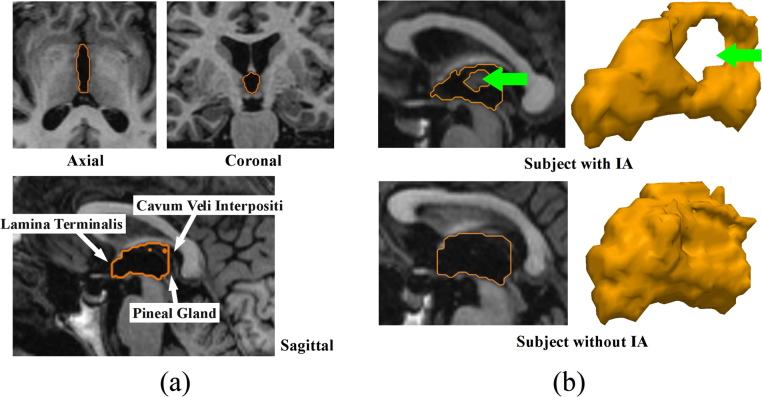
Third ventricle in structural magnetic resonance images. (a) small size and the low contrast of the surrounding structures (indicated by white arrows). Orange contours in the image are the third ventricle. (b) topological variations including the presence (above) and absence (below) of the inter-thalamic adhesion (IA). Green arrows indicate the holes of the third ventricle, where the IA passes through. (For interpretation of the references to color in this figure legend, the reader is referred to the web version of this article.)
